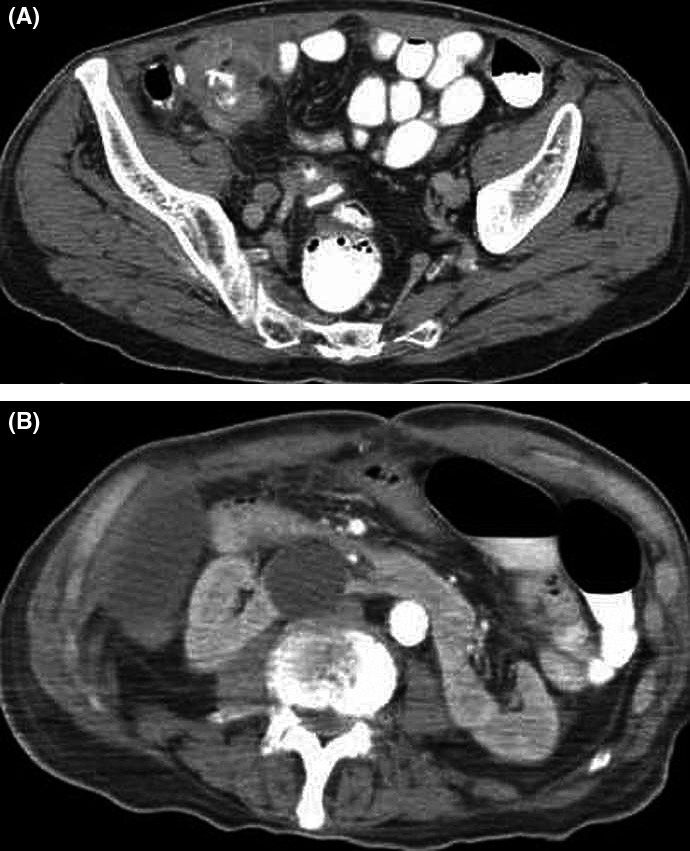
A, CT showed thickening of cecum wall with luminal narrowing. B, CT showed horseshoe kidney with a giant simple cyst at the isthmus of the renal fusion
